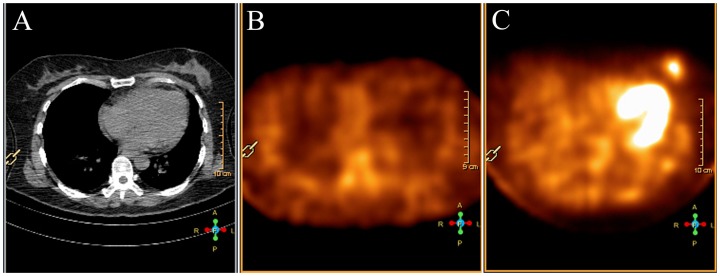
A 3.2 cm fibroadenoma of the left breast in a 47-year-old woman (Patient 30). (A) CT scan demonstrates a mass in the left breast. (B) ^99m^Tc-3P_4_-RGD_2_ SMM demonstrates low uptake of ^99m^Tc-3P_4_-RGD_2_ in the tumor (T/NT = 1.34). (C) ^99m^Tc-MIBI SMM demonstrates focal uptake of ^99m^Tc-MIBI in the tumor (T/NT = 2.81).
